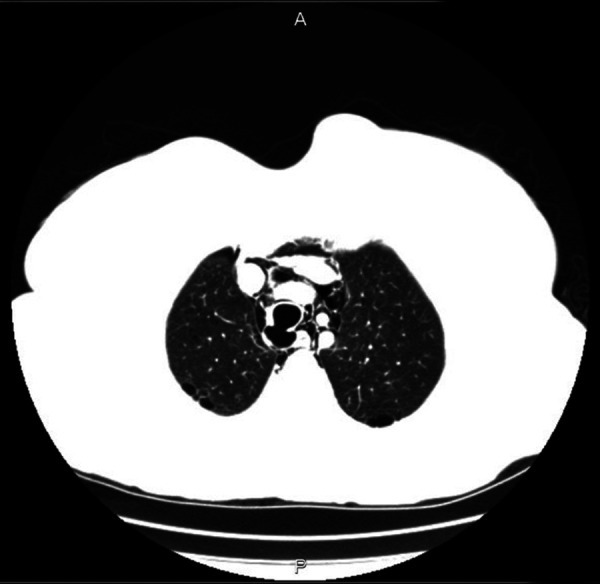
Computed tomography image of level IIIA PITL.
